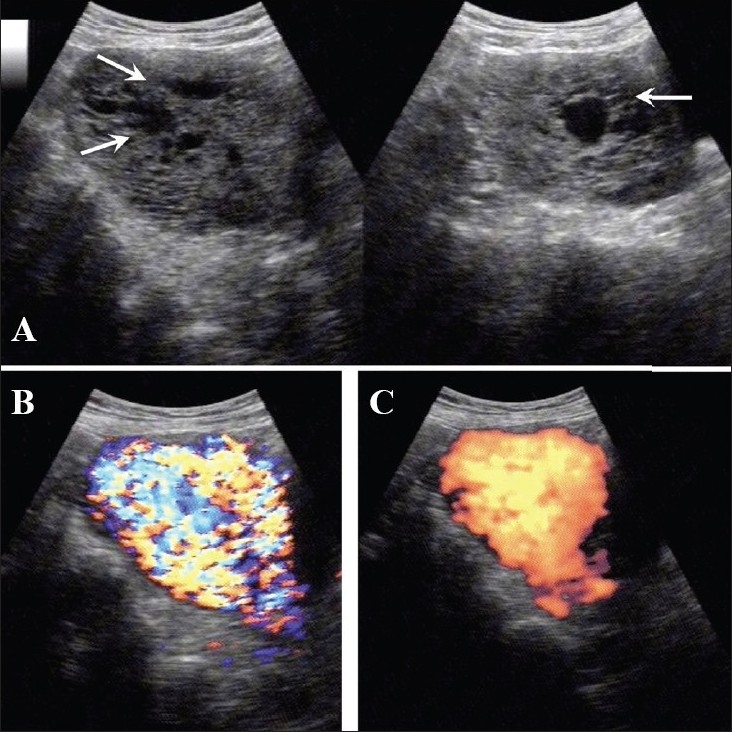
(A) Invasive mole. Extensive cystic foci (arrows in A) are seen in the uterine cavity; the lesion also shows intense vascularity on color Doppler (B) and almost complete filling of the uterus with color on power Doppler (C)
